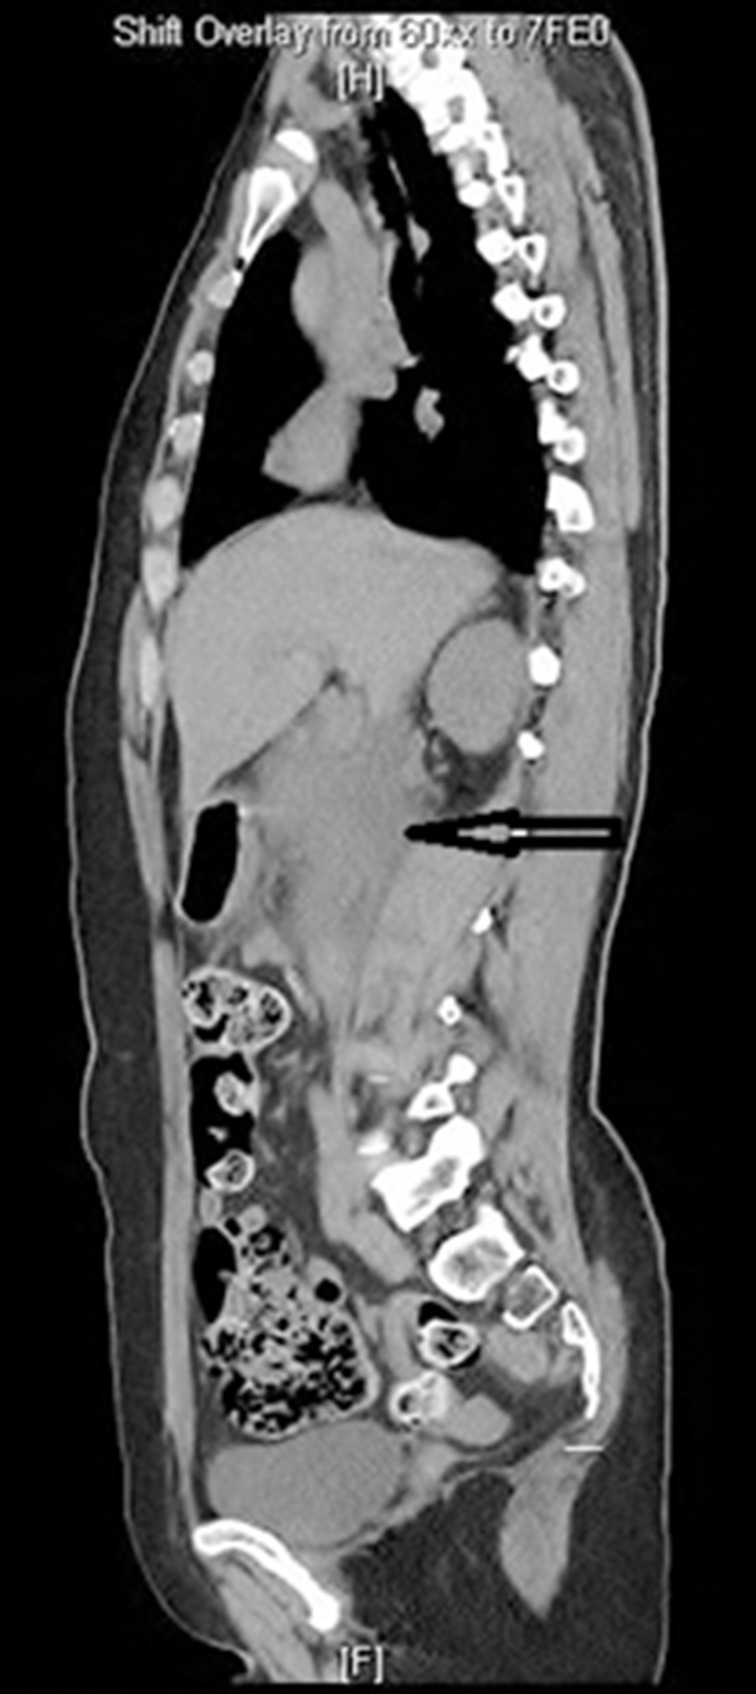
Sagittal axis computed tomography (CT) of the chest and abdomen on day of admission showing peripancreatic fluid in acute pancreatitis. The fluid extends into the right flank between the spleen and splenic flexure of the colon.
